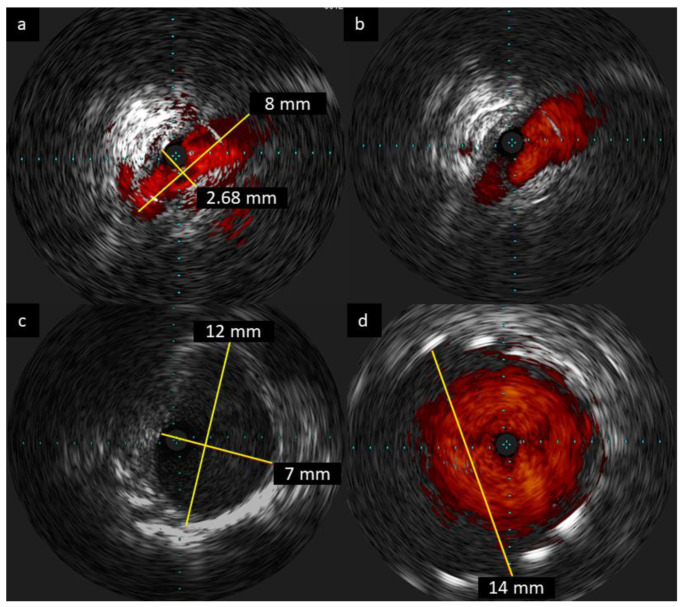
The intravascular ultrasound (IVUS) examination of right segmental A9/A10 pulmonary artery. Critical stenosis was confirmed in the first BPA session (a). The second BPA session revealed elastic recoil phenomenon after the use of increasing diameters (4.0 mm, 7.0 mm, and 8.0 mm) of balloon catheters (b). Distal A9/A10 reference diameter (c) for proper stent selection in third BPA session. Final result after 14 × 30 mm stent implantation (d).
